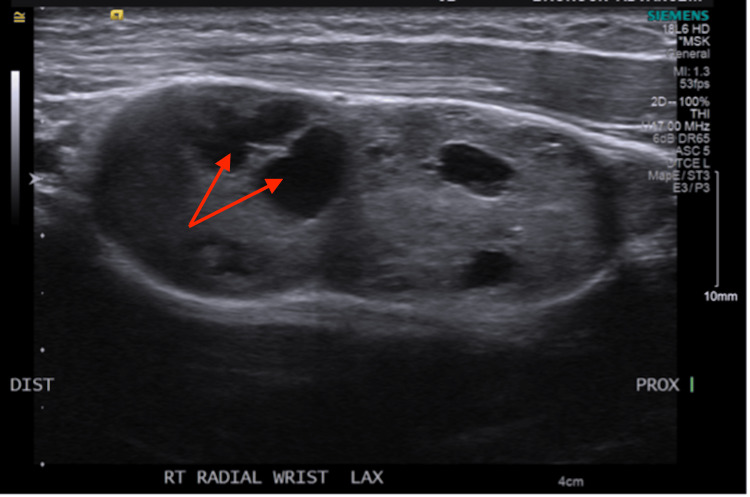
Ultrasound image showing a bilobed dumbbell-shaped mass in the distal left forearm. The mass is centered within the soft tissues, underlying the adjacent flexor tendons, and measures 2.8x1.4x2.0 cm proximally and 5.4x1.9x2.67 cm more distally. MRI, magnetic resonance imaging
